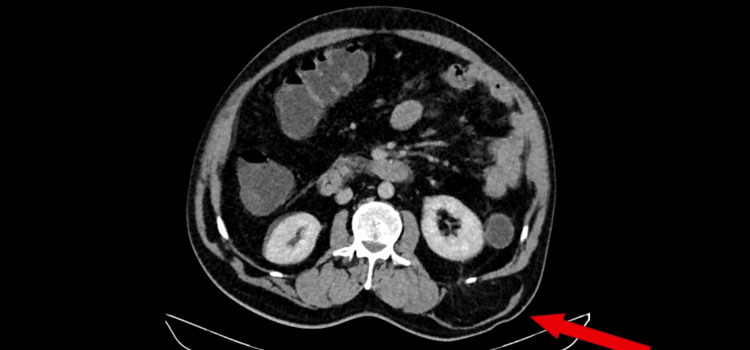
Left lumbar hernia defect seen in CT scan.
